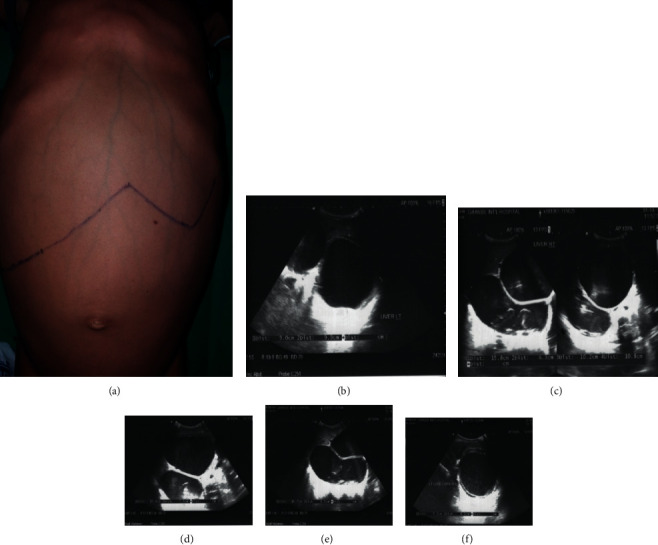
Massive hepatomegaly on clinical examination and ultrasonography showing 3 giant (≥ 10 cm) cysts involving both the liver lobes.
